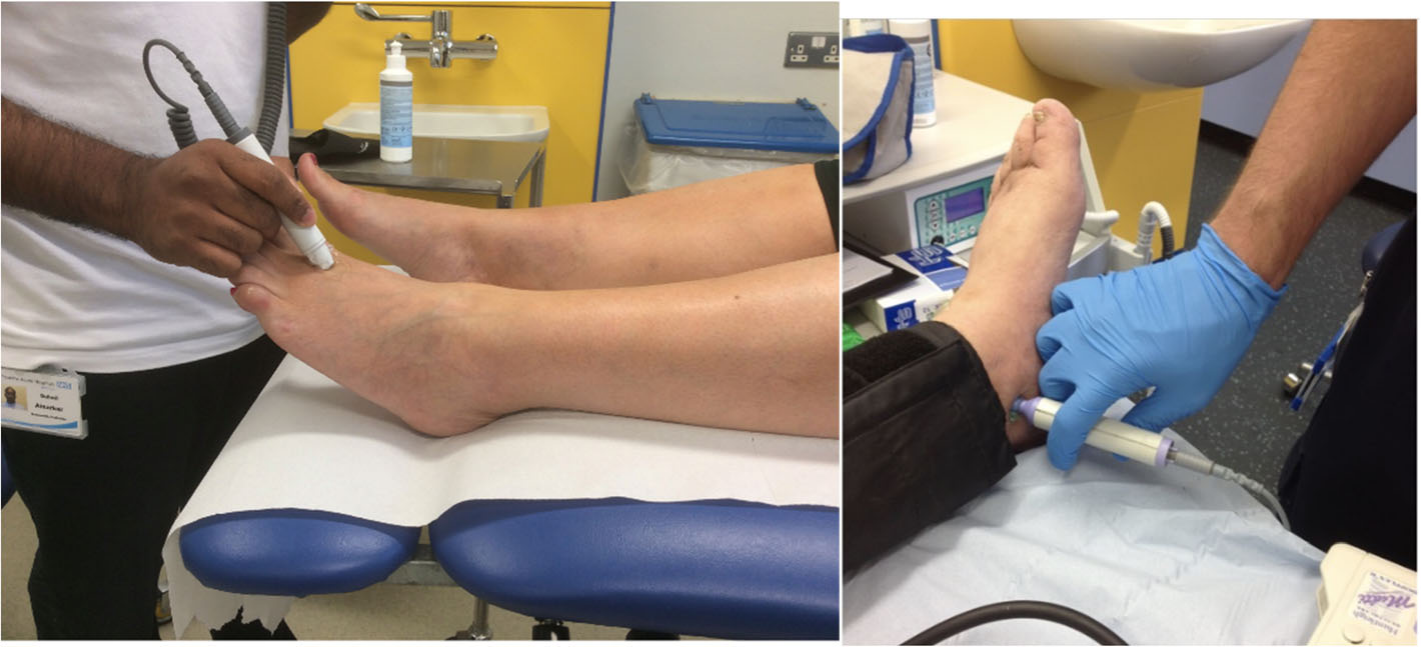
Image depicting anchored hand positioning for using Doppler on dorsalis pedis artery (L) and posterior tibial artery (R)
